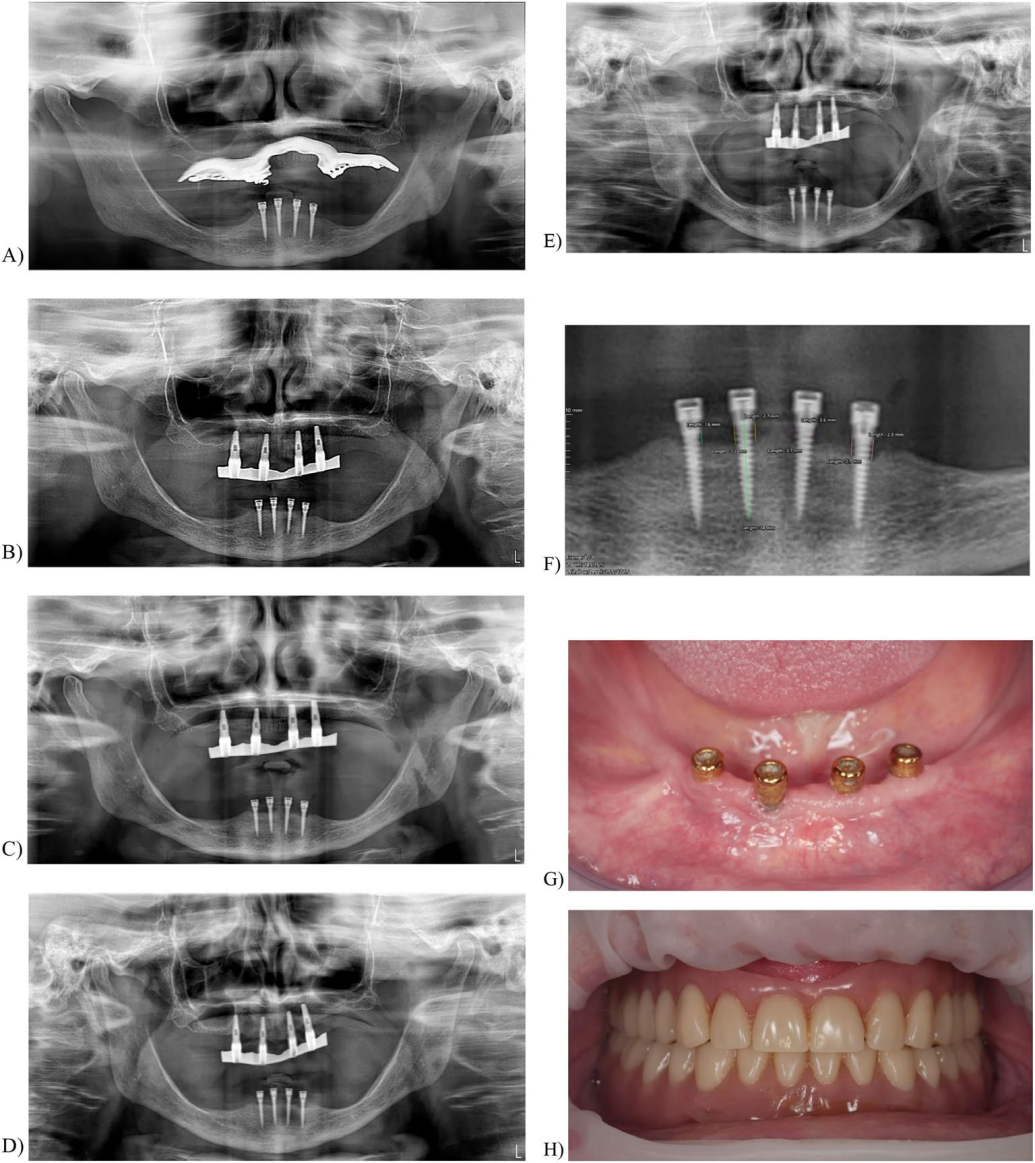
Example of a edentulous patient treated with four Biomax Lodi Locator Implant System. (A) Orthopantomography (OPG) showing the T0. (B) Orthopantomography (OPG) showing the T1. (C) Orthopantomography (OPG) showing the T5 (D) Orthopantomography (OPG) showing the T8 (E) Orthopantomography (OPG) showing the T10 (F) MBL calibration with Weasis Software (G, H) Clinical results.
